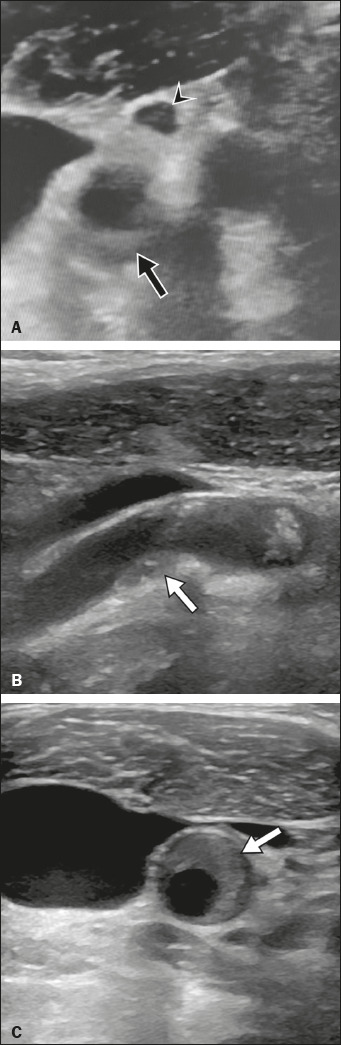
A: Grayscale carotid ultrasound image of a 67-year-old man
